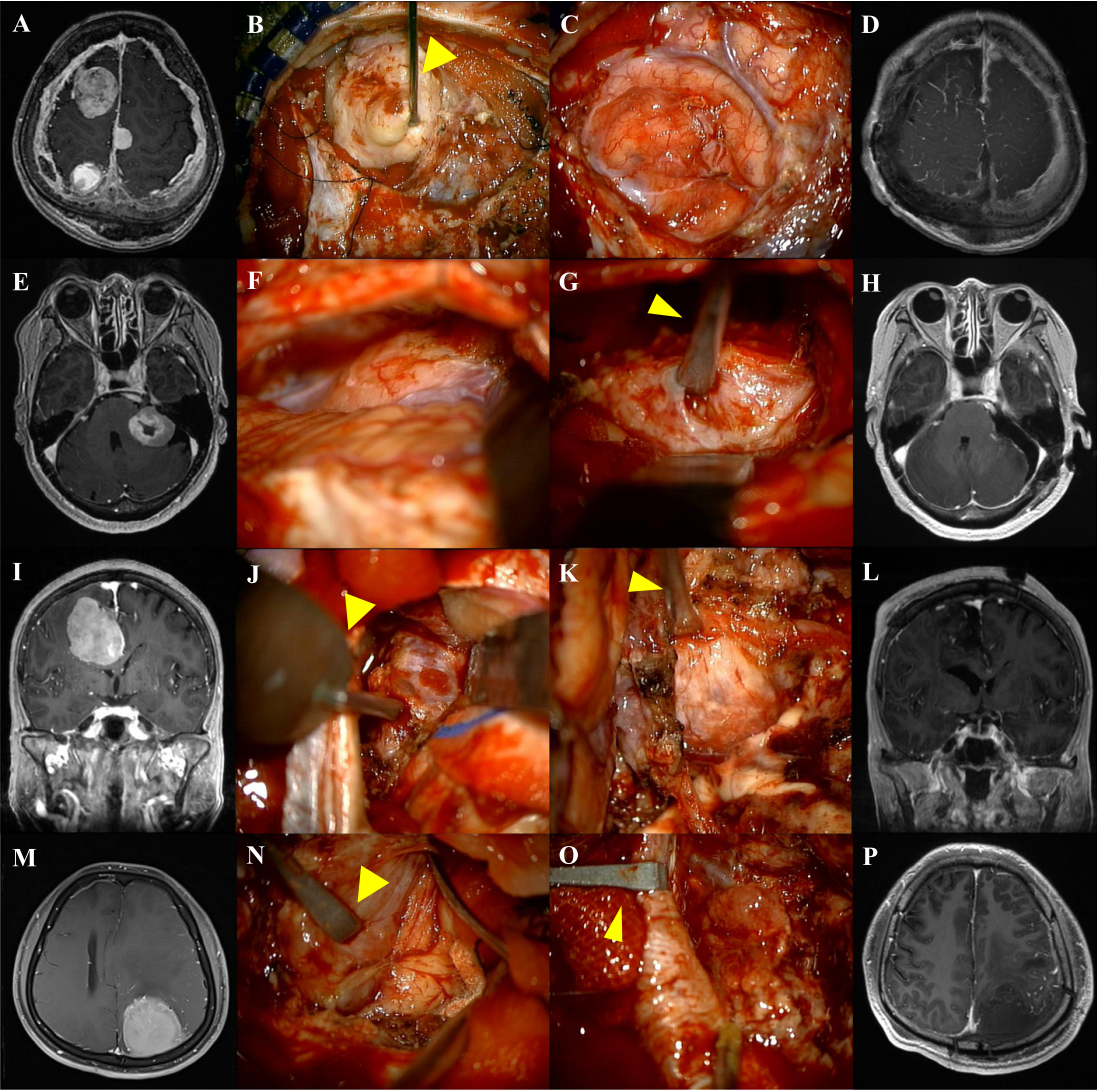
a, e, i, m Initial preoperative magnetic resonance (MR) image. b, c, f, g, j, k, n, o Intra-operative images showing the tumor retractor in place. d, h, l, p Postoperative gross total resection MRI images
